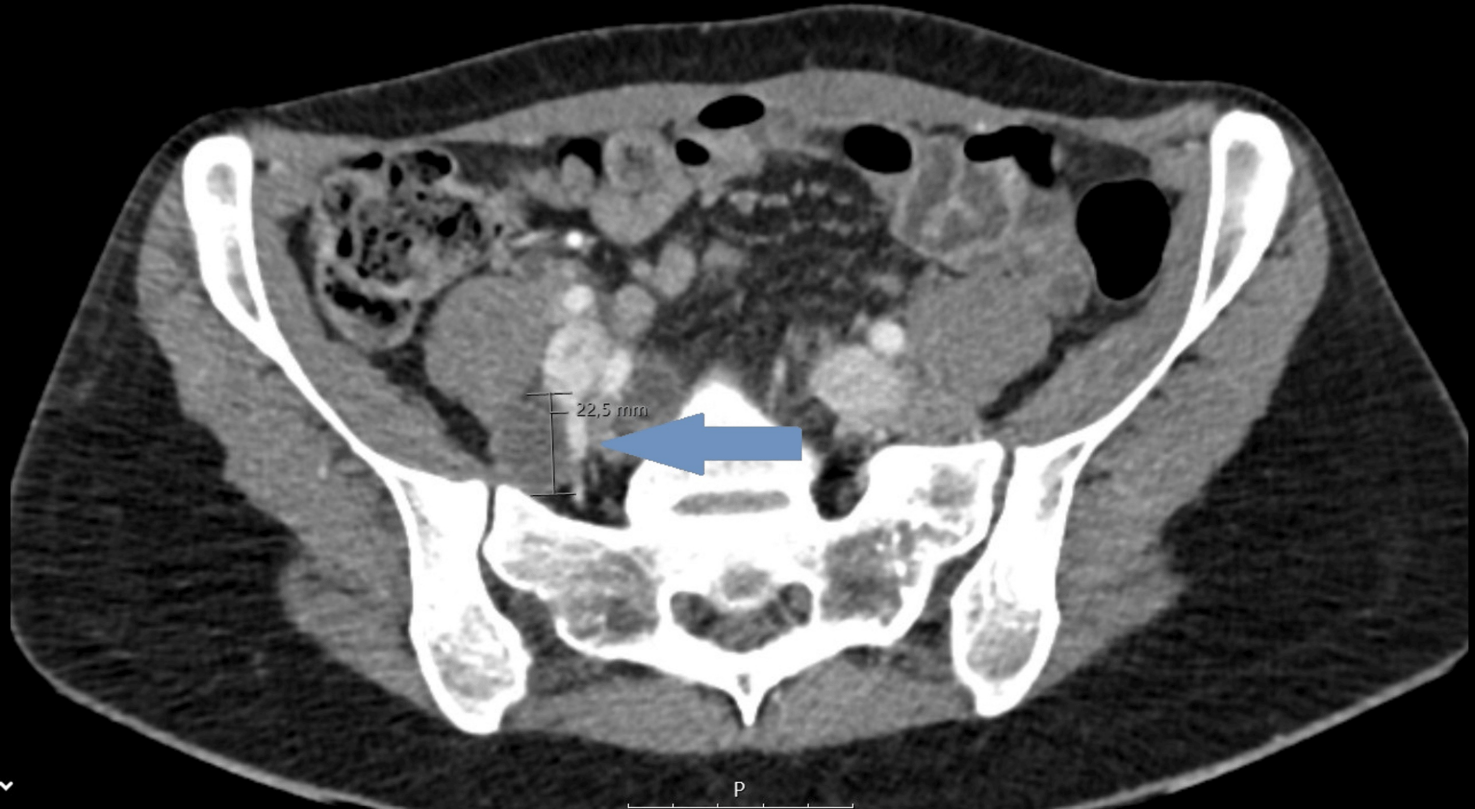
Abdominopelvic computed tomography scan showing a multiloculated cystic formation measuring 78 x 22.5 mm in the upper left retroperitoneum, suggestive of a lymphangioma (arrow).
